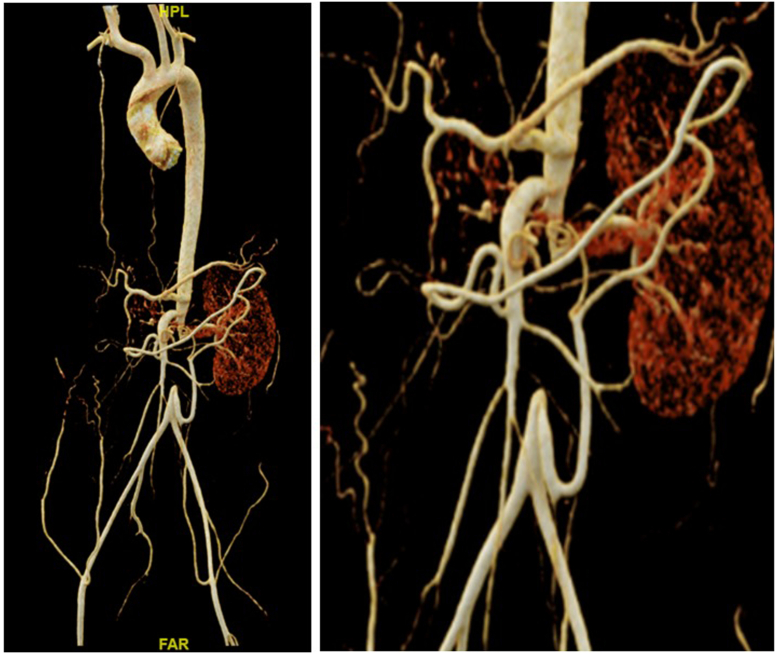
Volume Rendering Technique Computed Tomography Angiography Pre Percutaneous Transluminal Angioplasty in Distal Aortic Occlusion
